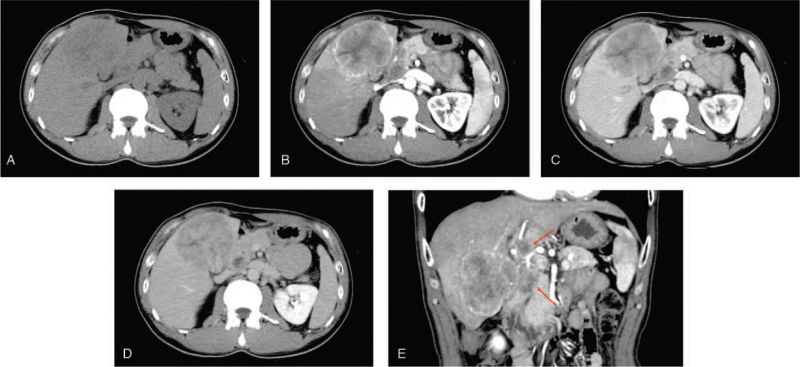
Contrast-enhanced computed tomography (CT) findings at the time of diagnosis. (A-D) Axial CT images in the (A) precontrast, (B) arterial, (C) portal venous, and (D) equilibrium phase. (E) Coronal contrast-enhanced CT image in the arterial phase. CT revealed a mass of 70 mm in size with indistinct margins occupying the anteromedial hepatic segments. Only the margin of this liver mass showed contrast enhancement. The gallbladder was surrounded by this liver mass, narrowing its lumen. Many swollen lymph nodes (red arrow) can be observed in the hepatoduodenal ligament.
